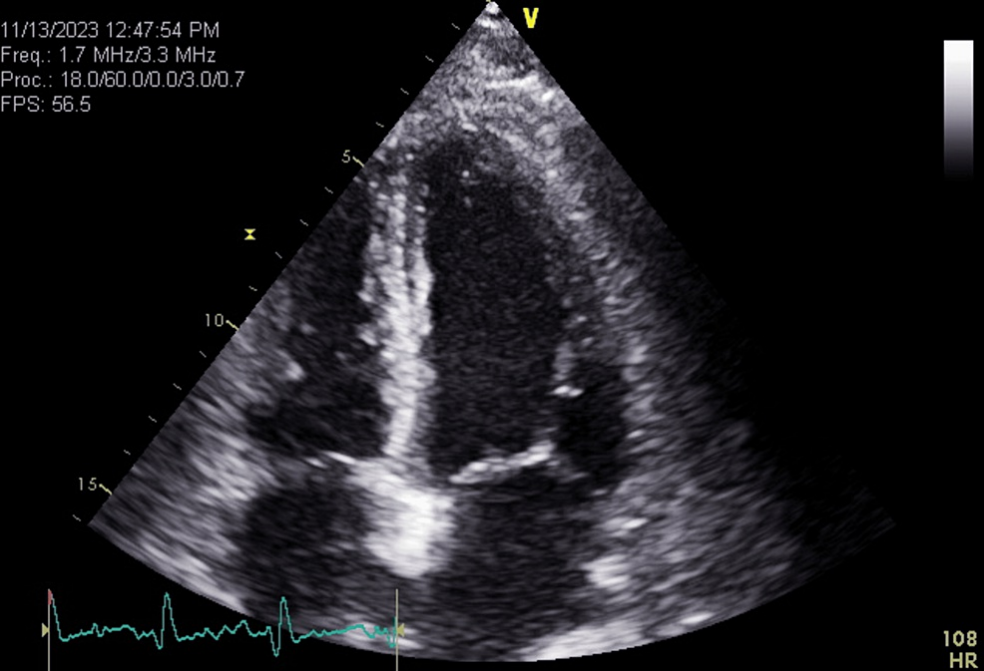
Official echocardiogram after pericardial fluid drain shows mild stable pericardial fluid in cardiac lab, Day 1 post pericardiocentesis
